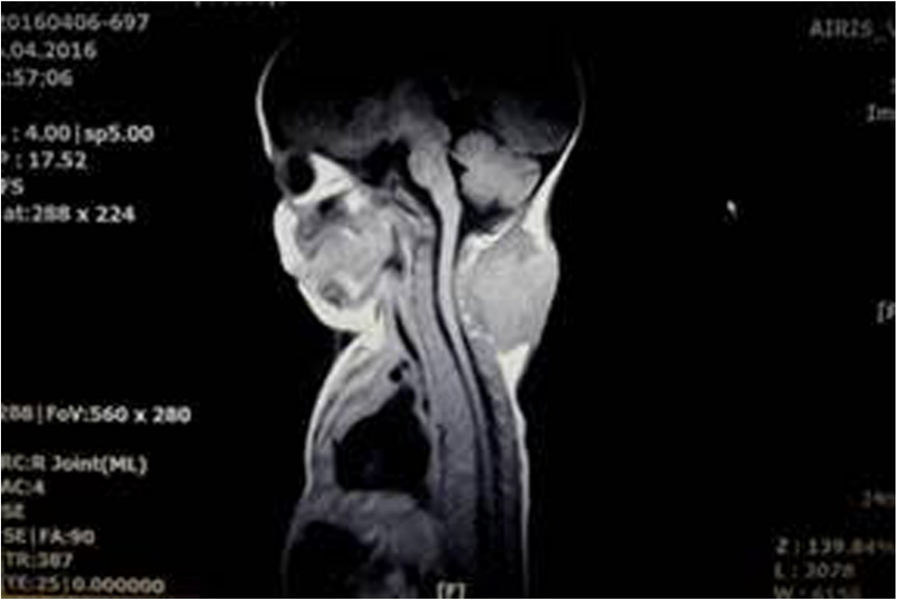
Magnetic resonance imaging of soft tissue mass in the neck – T1-weighted sequence
